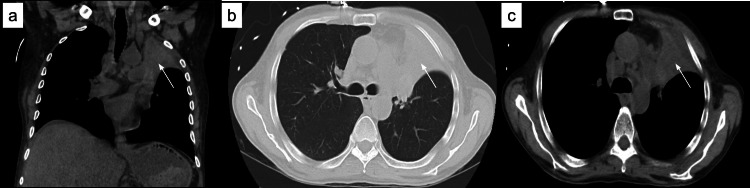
computed tomography (CT) chest without contrast revealed obstruction of the left upper lobe bronchus with the complete collapse of the left upper lobe (white arrow). (a-coronal view, b and c - axial view).
